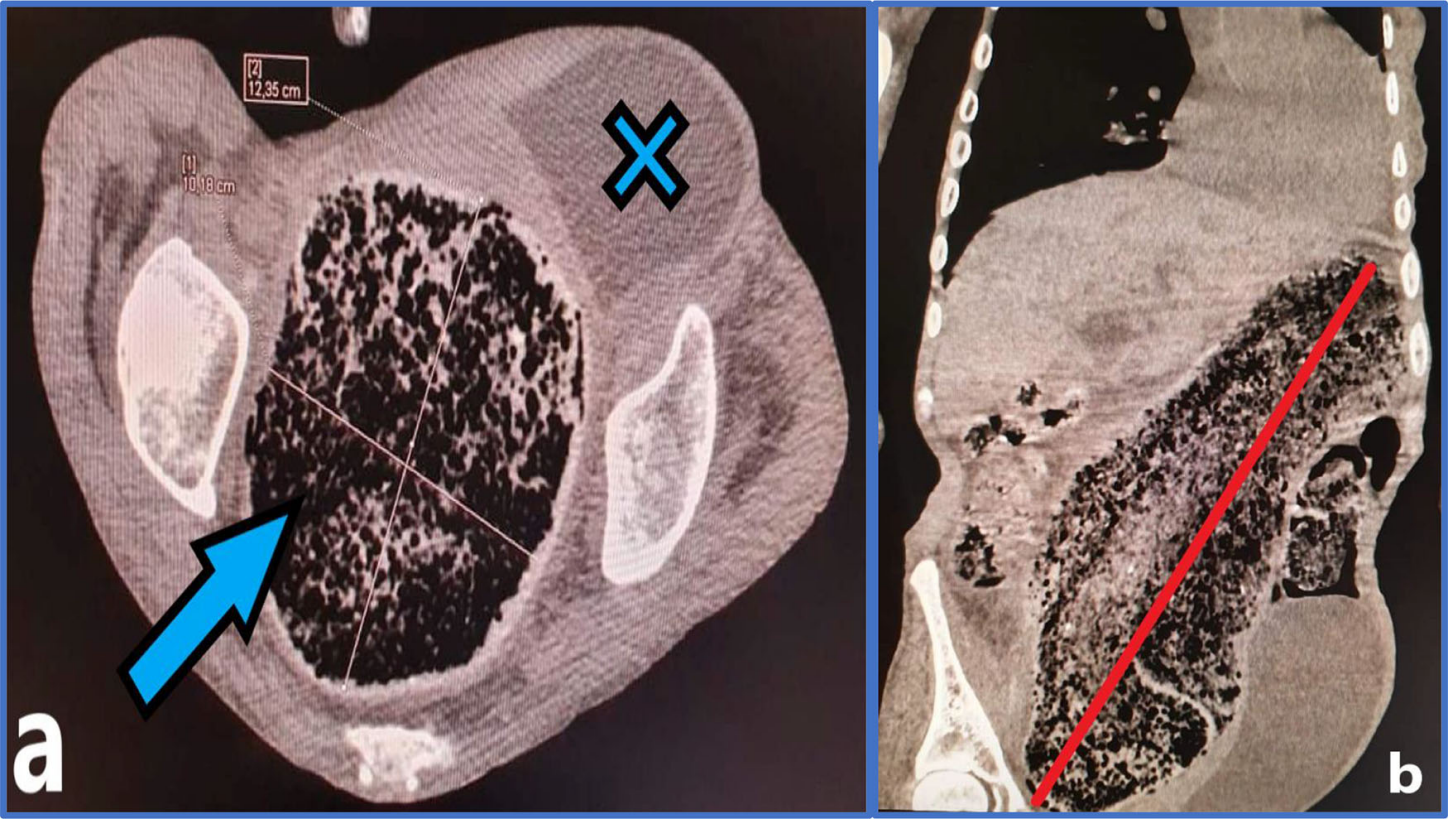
Abdominal computed tomography with an axial (a) and coronal (b) cuts revealing a distension of the rectum and sigmoid colon by a giant fecaloma of 10 × 12 cm (a, Blue arrow) going up to the left hypochondrium (b, Red line).
